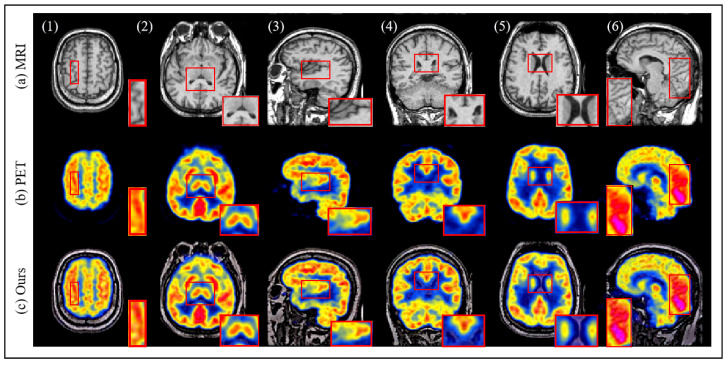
The results of image fusion on the Harvard dataset.The red box displays the texture details of the PET image, MRI image and the fused image.
